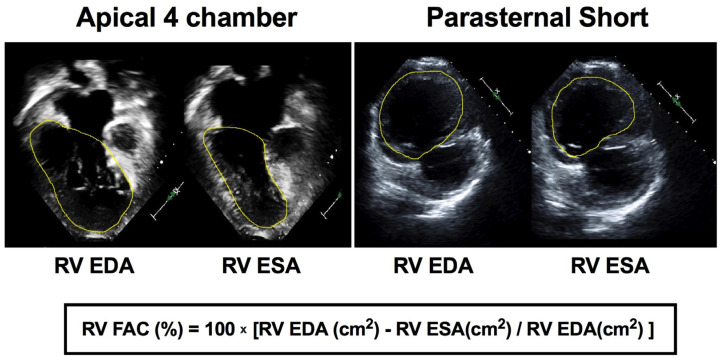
Fractional area of change (FAC). RV FAC was derived by tracing the area bound by endocardial borders of the right ventricle at end-diastole (EDA) and end-systole (ESA) in the apical four-chamber view and the parasternal short-axis view. RV FAC was calculated as 100 × EDA-ESA/EDA and expressed as a percentage.
